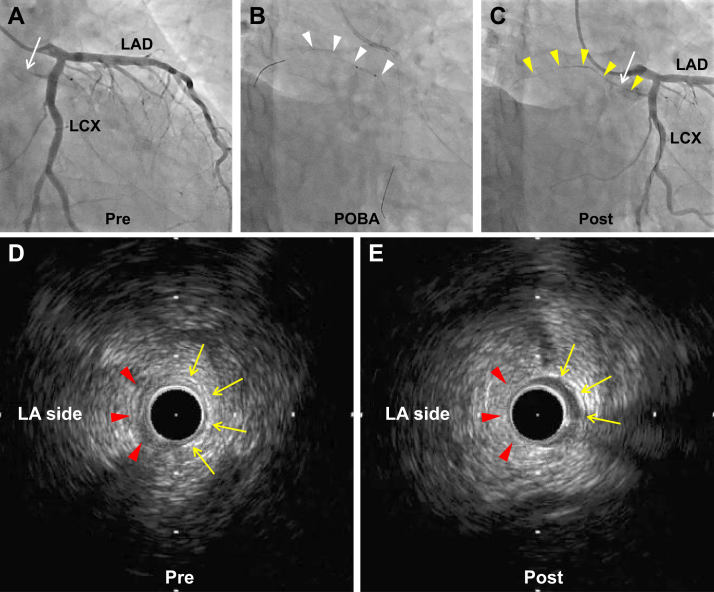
Percutaneous coronary intervention (PCI) for the occluded sinus nodal artery. A: Pre-PCI coronary angiography (CAG) showing the occluded sinus nodal artery (*white arrow*) originating from the left circumflex coronary artery (LCX). B: Plain old balloon angioplasty (POBA) using semi-compliant balloon (diameter = 1.5mm) for the occluded sinus nodal artery. *White arrowheads* indicate balloon-treated sites. C: Post-PCI CAG showing the recanalized sinus nodal artery (*yellow arrowheads*). D: Pre-PCI intravascular ultrasound (IVUS) cross-section showing the occluded lesion of the sinus nodal artery (corresponding with *white arrow* in panel A). The vessel diameter was approximately 1.5 mm and the lumen was invisible. The perimedial high-echoic band was partially identified (*yellow arrows*), while the vessel structure (3 layers of the vessel) on the left atrium (LA) side could not be clearly identified (*red arrowheads*). The orientation of the LA side was determined based on the location of the small side branches of the sinus nodal artery also identified on angiography. E: Post-PCI IVUS cross-section showing the recanalized lumen of the sinus nodal artery (corresponding with *white arrow* in panel C). The vessel structure was partially identified (*yellow arrows*), although the structure on the LA side remained to be determined (*red arrowheads*). The echogenicity of the adventitia on the LA side was relatively low. LAD = left anterior descending artery.
